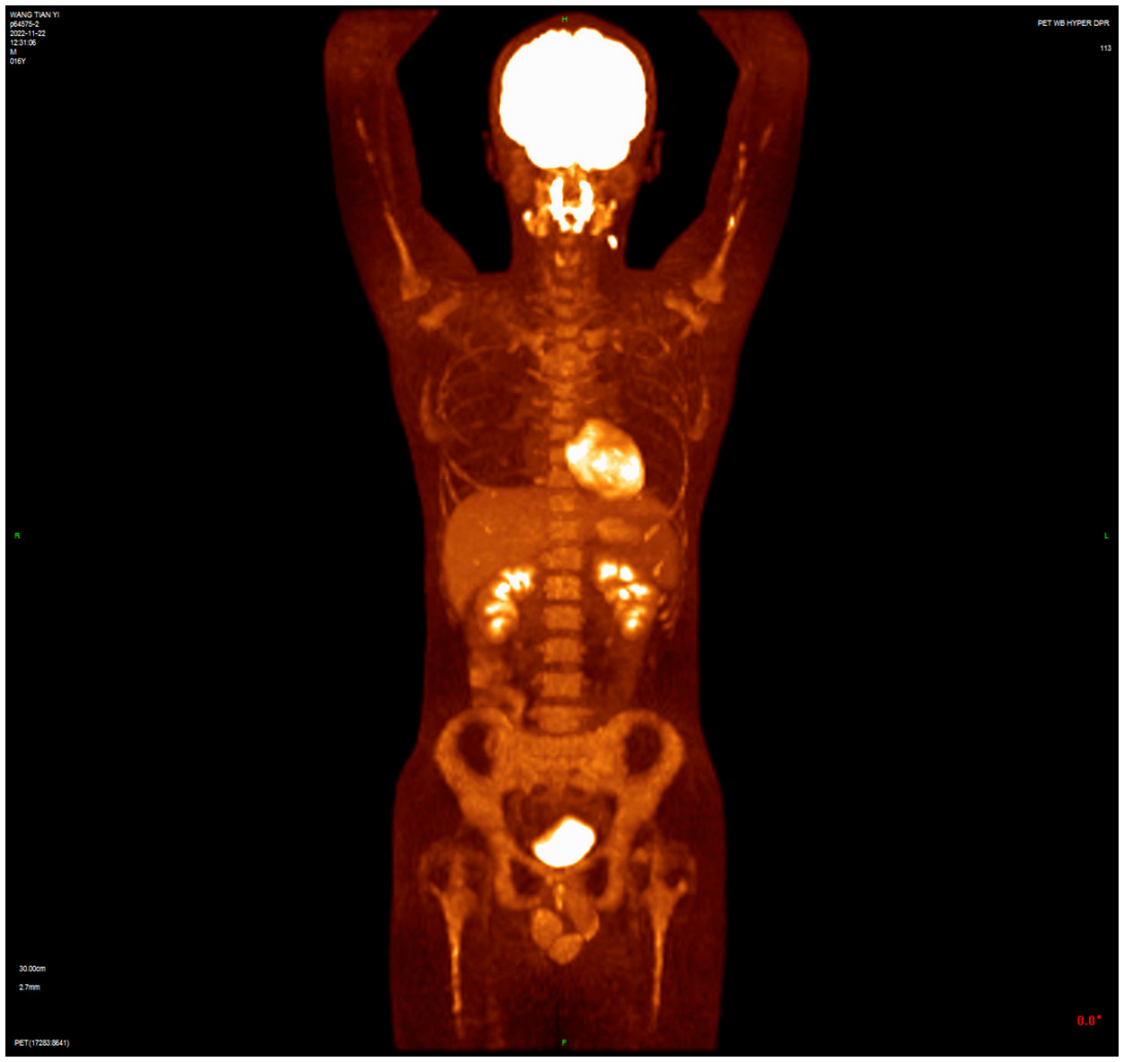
PET/CT after two cycles of treatment showed incomplete resolution of the lesion, with increased lymph node uptake of 18F-FDG in the left posterior cervical triangle.
